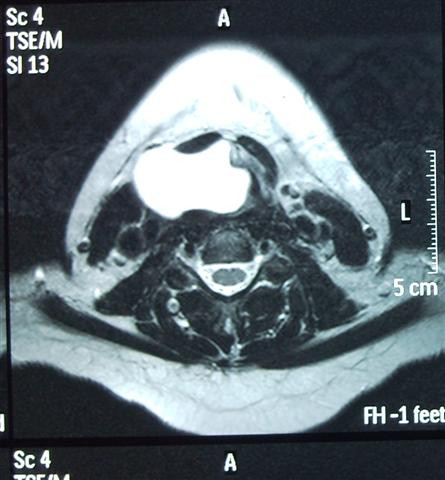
Sagittal T2-weighted MRI image of the neck demonstrating a well defined lesion.
